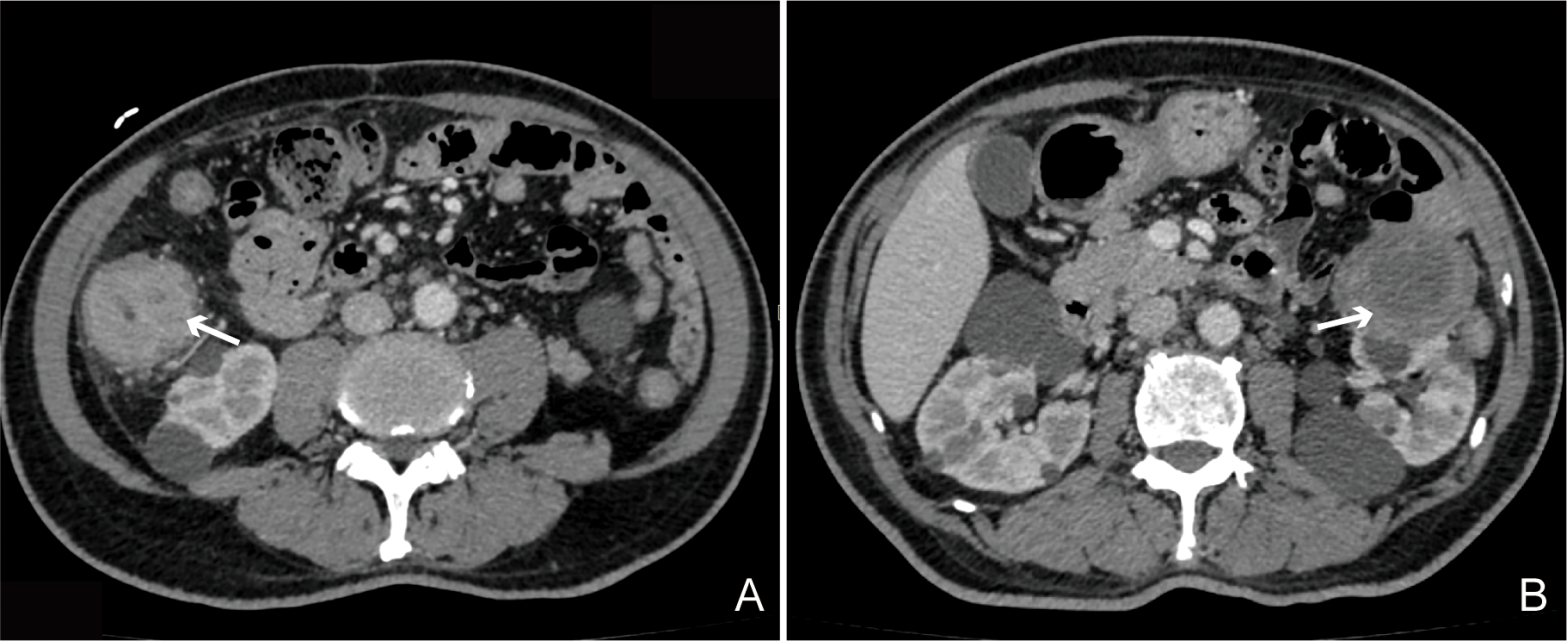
The abdominal CT scan demonstrating the presence of an ascending colon mass (A) and solid renal mass (B).
